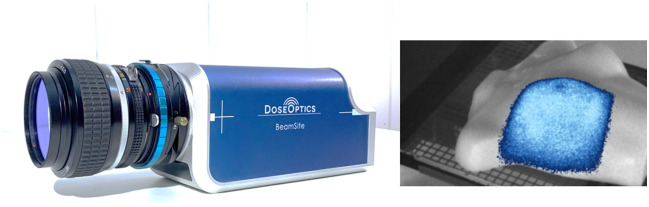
DoseOptics BeamSite camera technology (left) leverages detection of Cherenkov light (right)—emitted when high-energy x-ray beams interact with tissue—to visualize treatment dynamics in real time. Image courtesy of DoseOptics.
